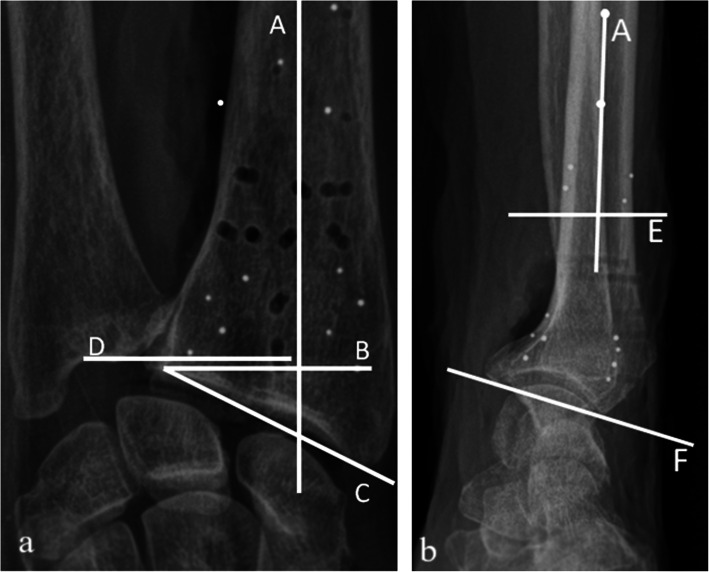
Radiographic measurements. Posterior-anterior projection (a); lateral projection (b). Line A represents the longitudinal axes of the radius (in both planes), determined by connecting two points (more than 2 cm apart) in the centre of the radial shaft. Line B is drawn from the most distal ulnar palmar corner of the radial articular surface and is perpendicular to line A. Line C connects the most distal ulnar palmar corner of the radial articular surface to the most distal part of the radial styloid tip. Line D abuts the most distal point of the articular surface of the ulna; it is parallel to line B thus perpendicular to the longitudinal axis of the radius. Line E is drawn at a convenient level perpendicular to the central long axis of the radius. Line F connects the most distal dorsal and palmar margins of the radial articular surface. The *ulnar variance* is defined as the length of the ulna relative to the radius, *i.e.*, the distance (mm) between lines D and B. The *radial inclination* is the inclination of the distal radial articular surface in the coronal plane, *i.e.*, the angle between lines B and C. *Tilt* describes the angulation of the distal radial articular surface in the sagittal plane, *i.e.*, the angle between lines E and F
